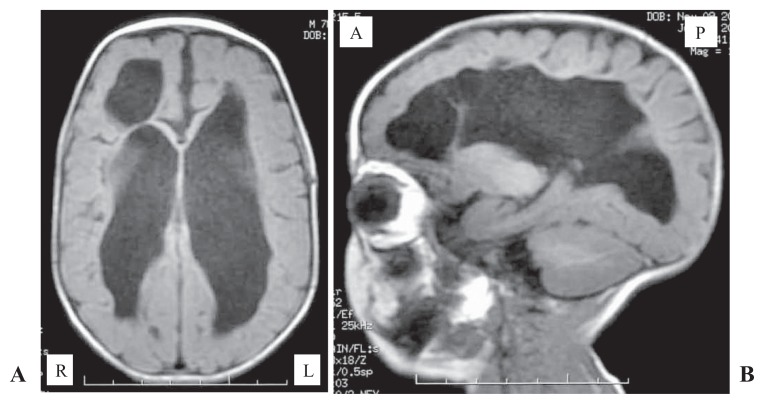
Brain magnetic resonance imaging performed on day 217. A: Axial T_1_-weighted section showing enlargement of lateral ventricles with a frontal large cyst. B: Parasagittal T_1_-weighted section showing a distinct enlargement of lateral ventricle and global reduction of white matter volume.
